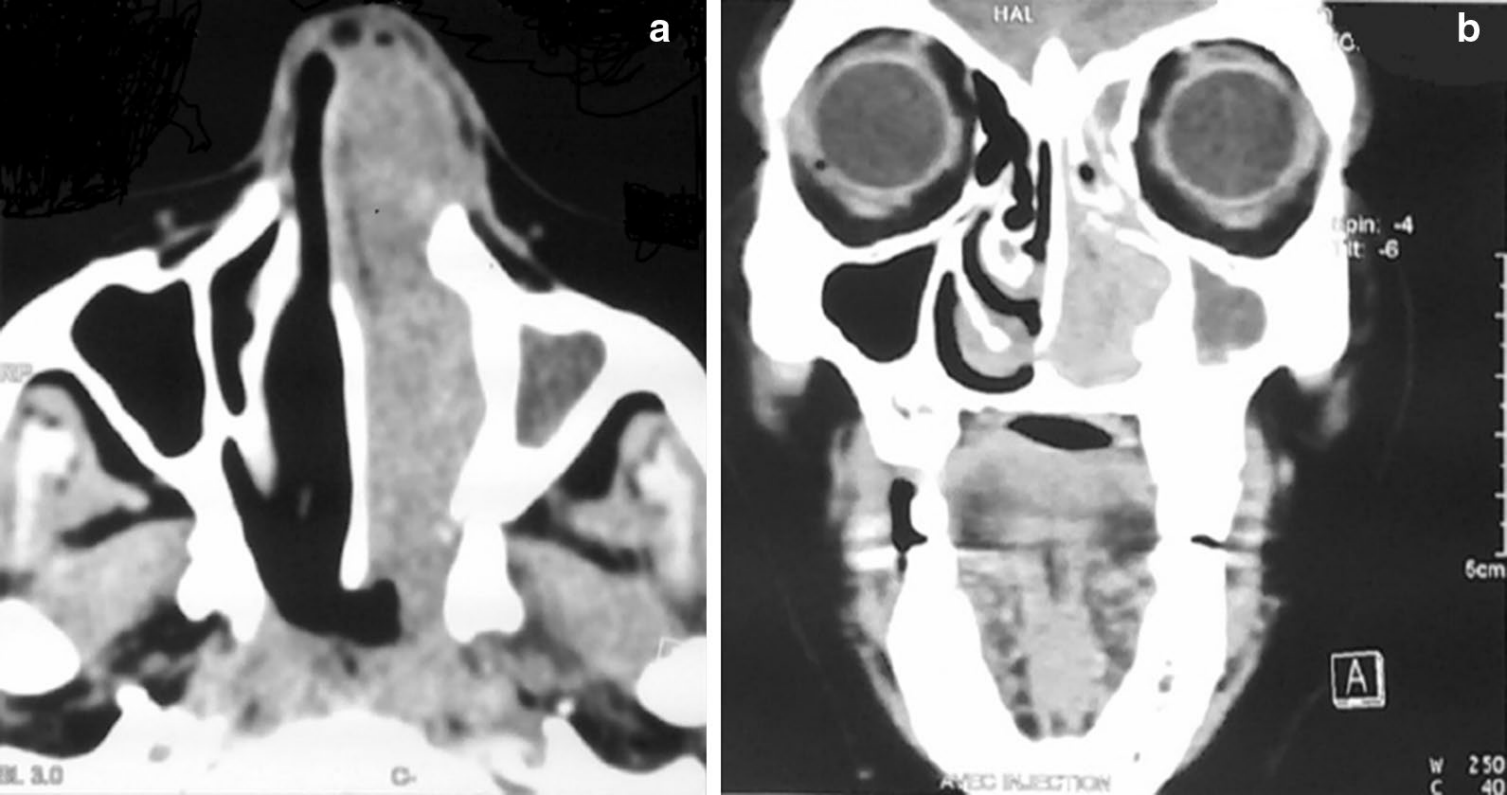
a Axial CT, b Coronal CT: demonstrated a tumor occupying the left nasal cavity causing erosion of nasal septum and extending posteriorly to the left choana and nasopharynx
